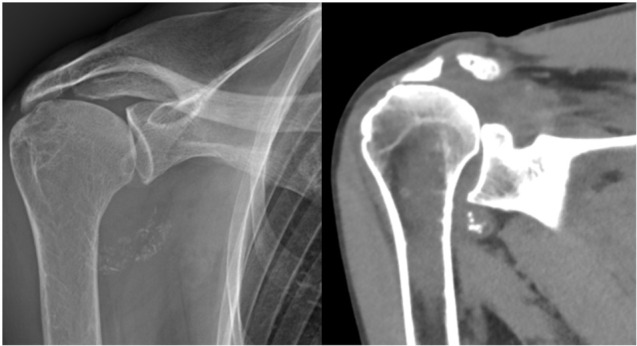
X-ray (left) and computed tomography (CT) scan (right) of the right shoulder showing well-defined calcifications in the axillary recess.
